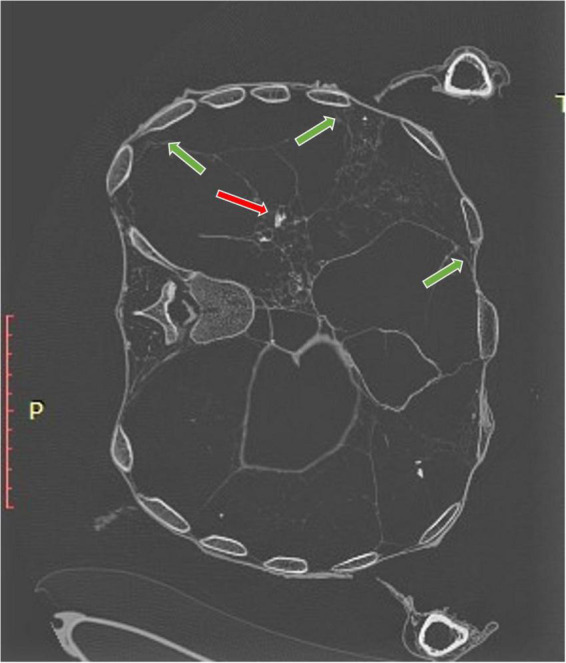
CT scan of the thorax: the lung parenchyma is focally condensed and shows multiple small calcifications (red arrow). Furthermore, both lungs appear as widely attached to the thorax wall (green arrows) suggesting post-inflammatory pleuritic adhesions.
